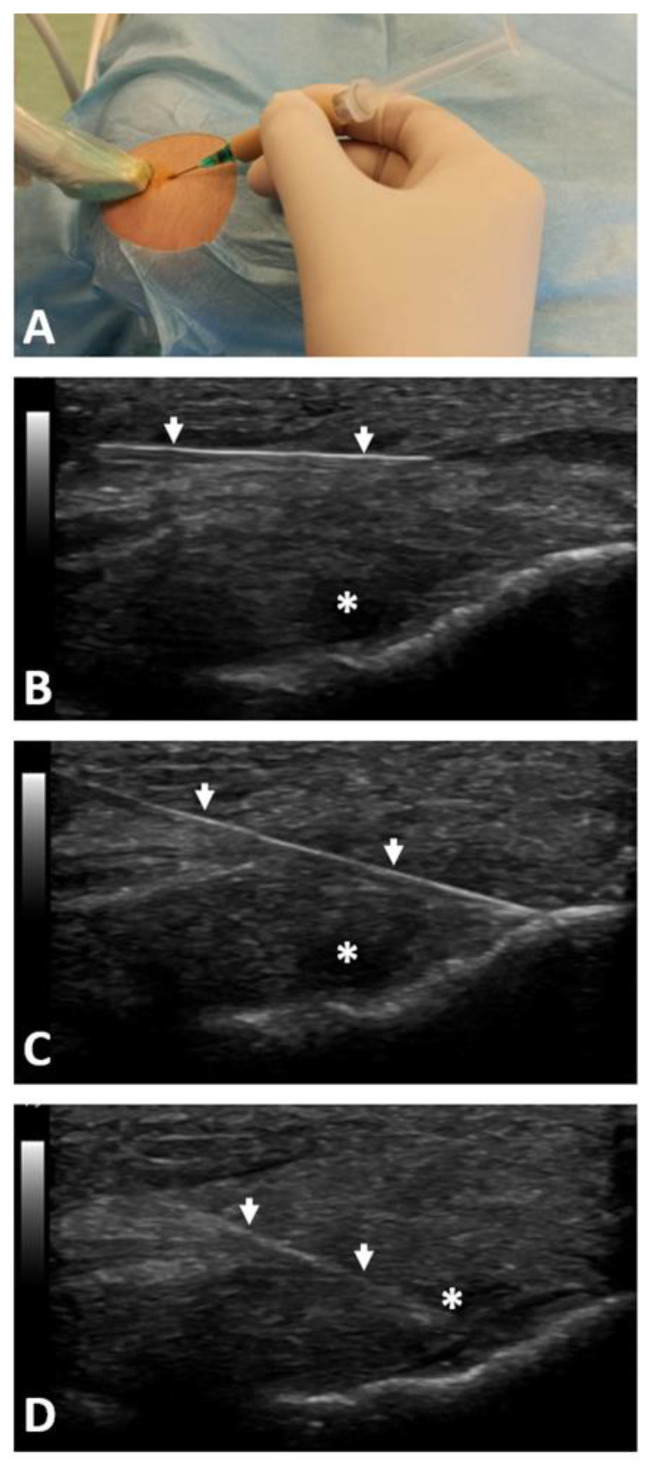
Presentation of ultrasound-guided PRP injection technique into common extensor tendon: (A) point of skin puncture with a needle guided by ultrasound probe in sterile conditions; (B) injection of 1–2 mL of 1% lidocaine in the subcutaneous region; (C) the peppering technique with multiple needle punctures through the fascia to the periosteum; (D) PRP injection into the hypoechogenic region of ECRB. PRP—platelet-rich plasma; ECRB—extensor carpi radialis brevis; arrows—view of the needle position under ultrasound; *—hypoechogenic region of the tendon.
